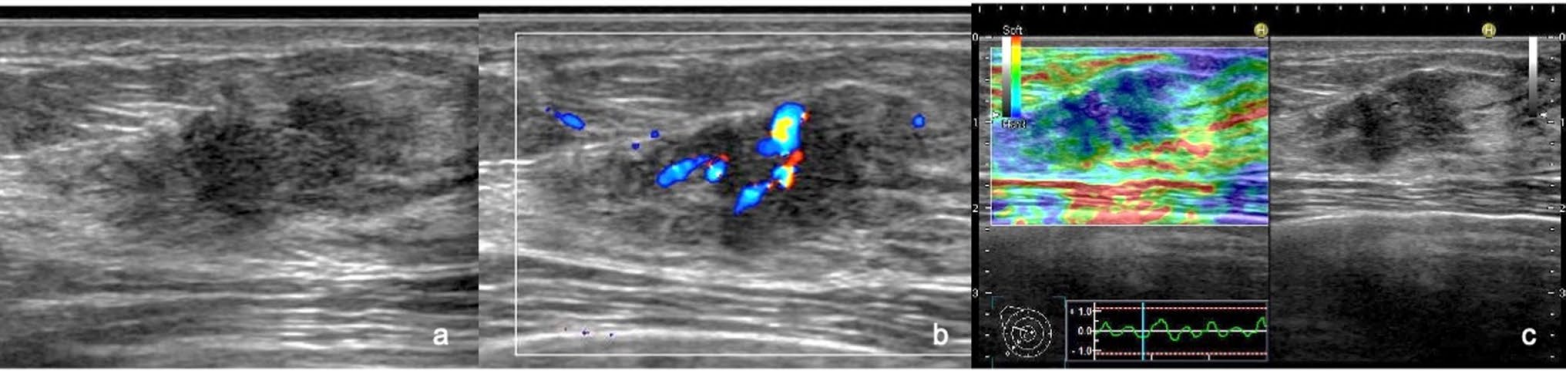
B-mode ultrasonography (US), color Doppler US, and elastography in a case of invasive lobular carcinoma. a A focal hypoechoic area in the mammary gland was seen on B-mode US. b It demonstrated vascularity of (2 +) on color Doppler US. c An elasticity score of 3 was assigned on elastography
